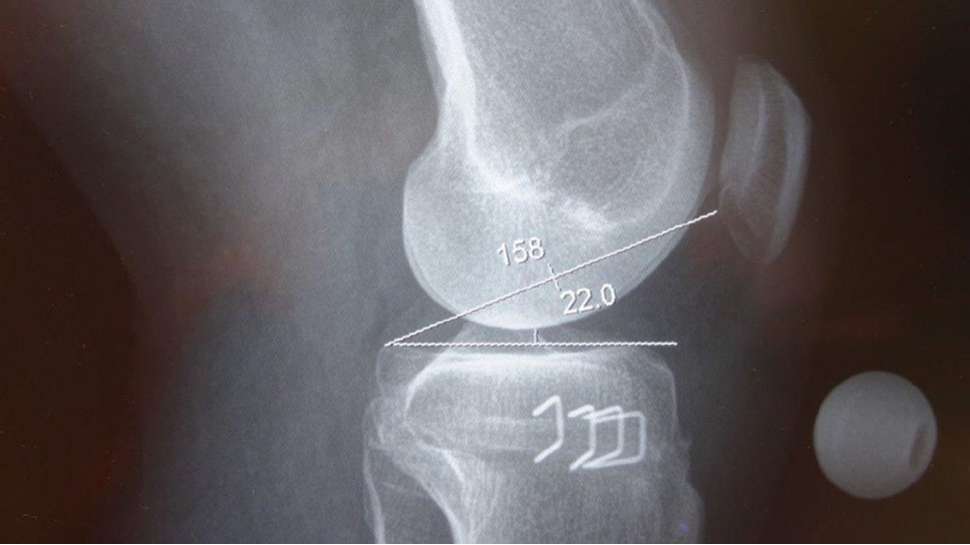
The postoperative plateau-patella angle measured 22° on the radiograph. Before surgery, it was 21.6°.
